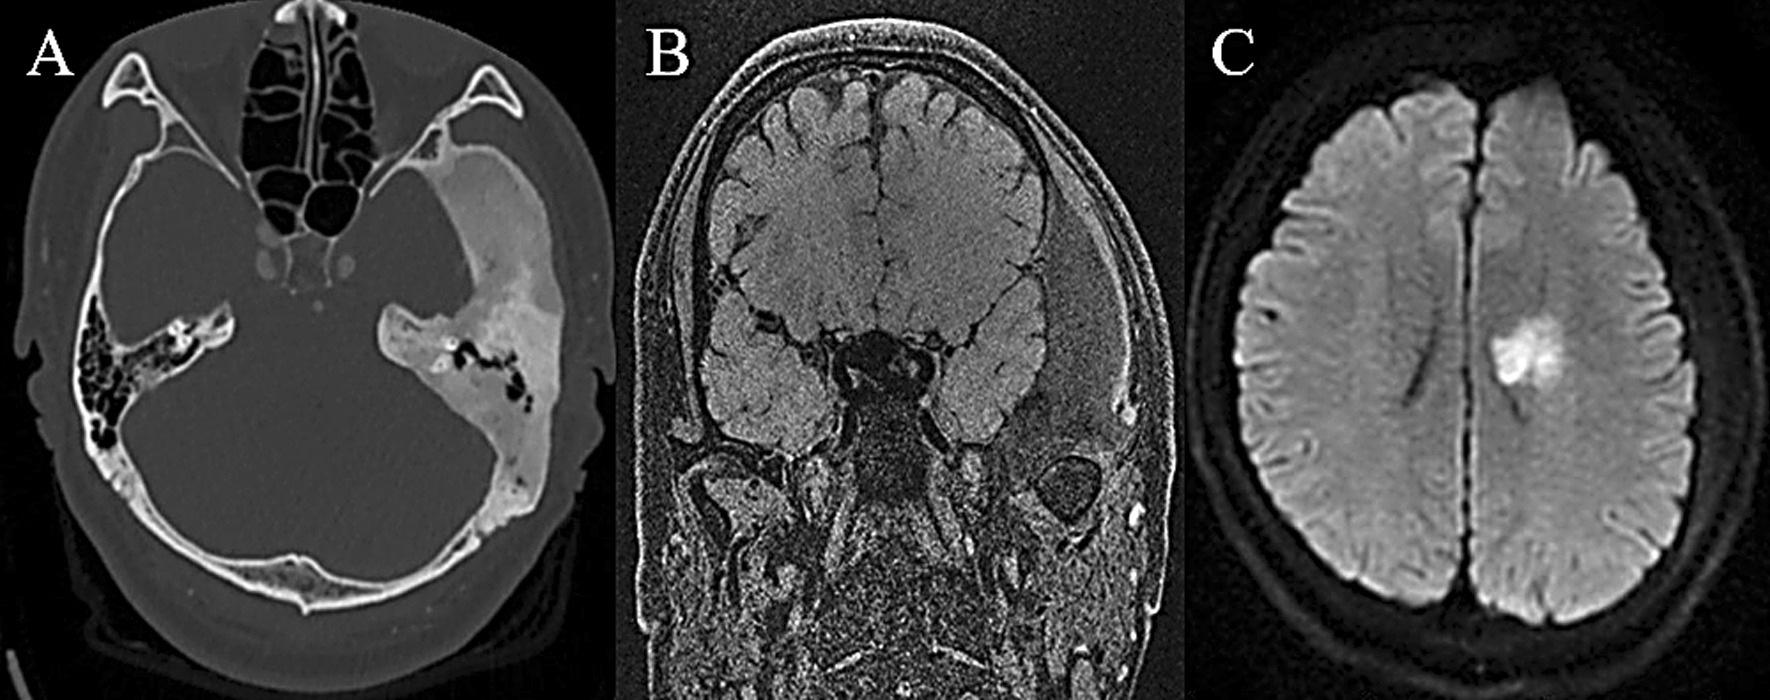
CT showing FD of the left parietal bone and temporal bone (A). MRI showing compression of the brain tissue (B) and acute cerebral ischemia of the left basal ganglia (C)
